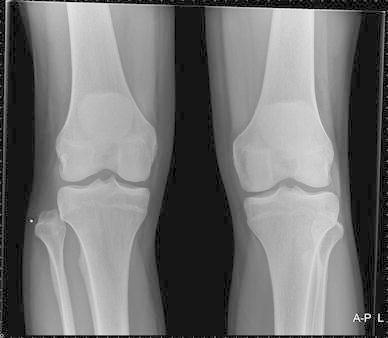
Bilateral AP X-ray of the knees. The aberrant position of the proximal fibula on the right side is evident when compared to the left knee. The direction of the luxation is anterolateral
